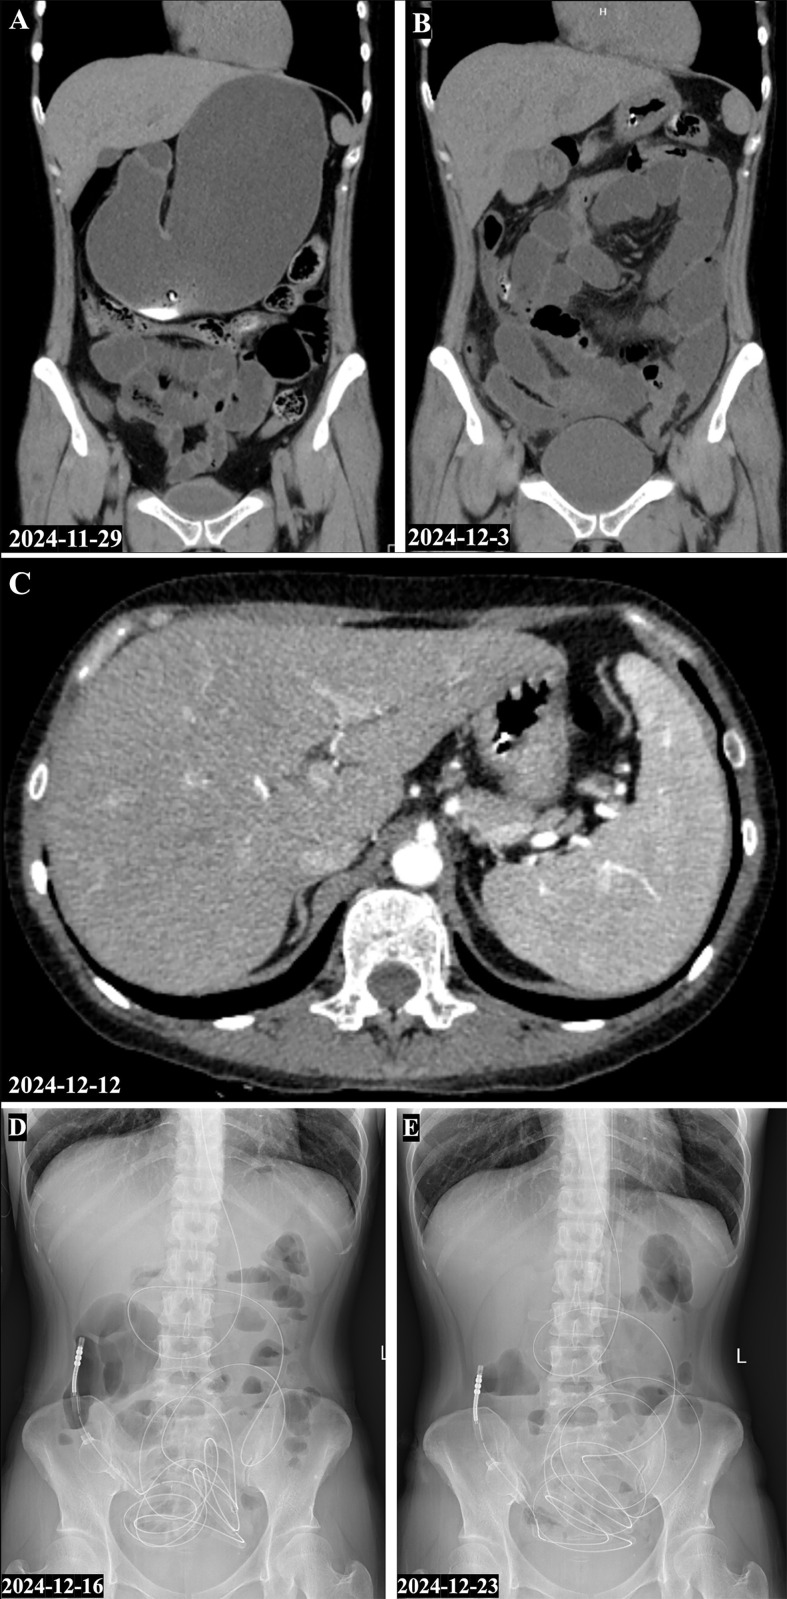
Serial imaging showing evolution and partial treatment response of intestinal pseudo-obstruction in systemic lupus erythematosus. (A) Abdominal and pelvic CT performed in the emergency department on November 29, 2024, showing massive gastric dilatation and intestinal distension. (B) Repeat CT on December 3, 2024, in the gastrointestinal surgery unit revealed progression of small-bowel dilatation. (C) Contrast-enhanced abdominal CT on December 12, 2024, demonstrated diffuse esophageal dilatation. (D, E). Upright abdominal plain films from December 16 and December 23, 2024, following intravenous methylprednisolone 40 mg/day, continued to show prominent air-fluid levels and intestinal gas accumulation without significant improvement.
